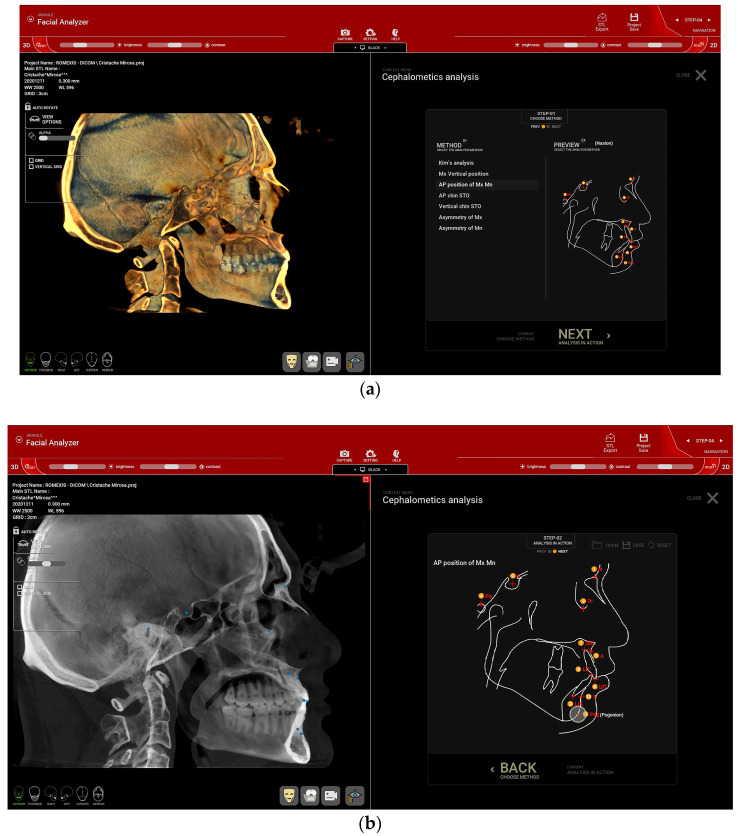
Cephalometric analysis of the CBCT in the R2Gate™ v 2.0 software. (a) The “AP position of Mx Mn” (antero-posterior position of the maxilla and mandible) option was selected. (b) Red dots within the image on the right (corresponding to the blue dots on the sagittal view of the patient’s CBCT) highlight the landmarks used, in the following order: nasion (N), sella (S), orbitale (Or), subspinale (A), upper incisor root apex (UIA), upper incisor incisal edge (UIT), lower incisor incisal edge (LIT), lower incisor root apex (LIA), supramentale (B) and pogonion (Pog).
